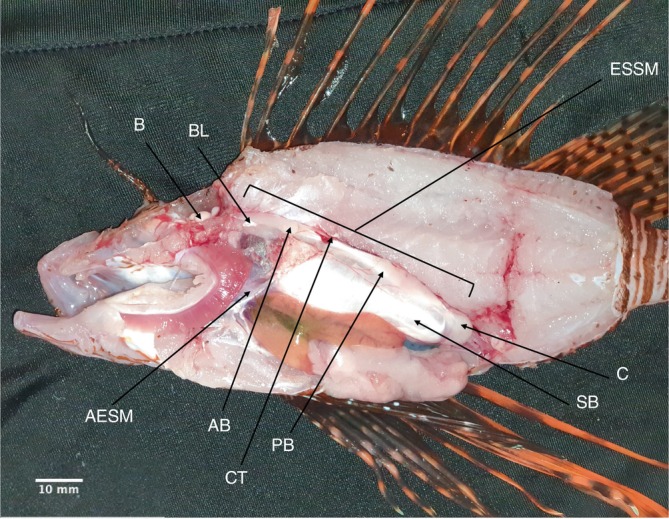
A cross‐section of an Indo‐pacific lionfish (*Pterois volitans*, TL 151.2 mm, sex unknown) showing (left to right, top to bottom) the brain (B), Baudelot's ligament (BL), extrinsic sonic swimbladder muscle (ESSM), the anterior extrinsic sonic muscle (AESM), the anterior belly of the extrinsic sonic swimbladder muscle (AB), the connective tissue between the bellies of the extrinsic sonic swimbladder muscle (CT), the posterior belly of the extrinsic sonic swimbladder muscle (PB), the swimbladder (SB) and the swimbladder cap (C), where the ESSM attaches posteriorly to the swimbladder.
